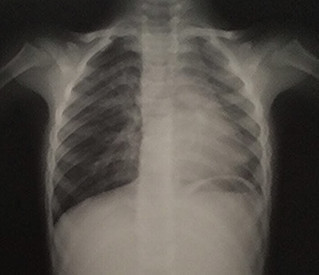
Radiographie thoracique montrant une asymétrie de taille des deux champs pulmonaire avec un foyer basal
